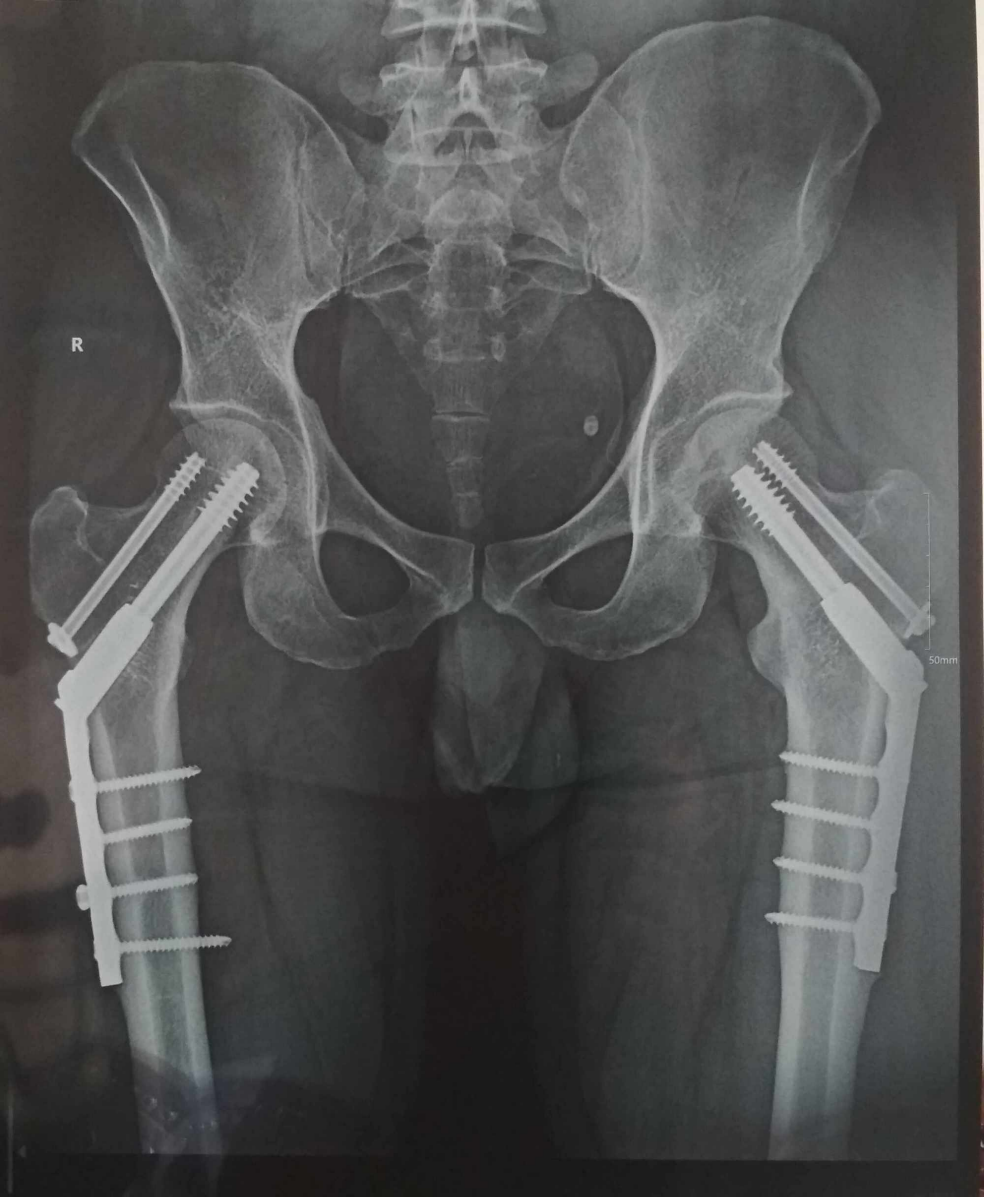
Radiograph at two years showing well united fractures without any complications.
